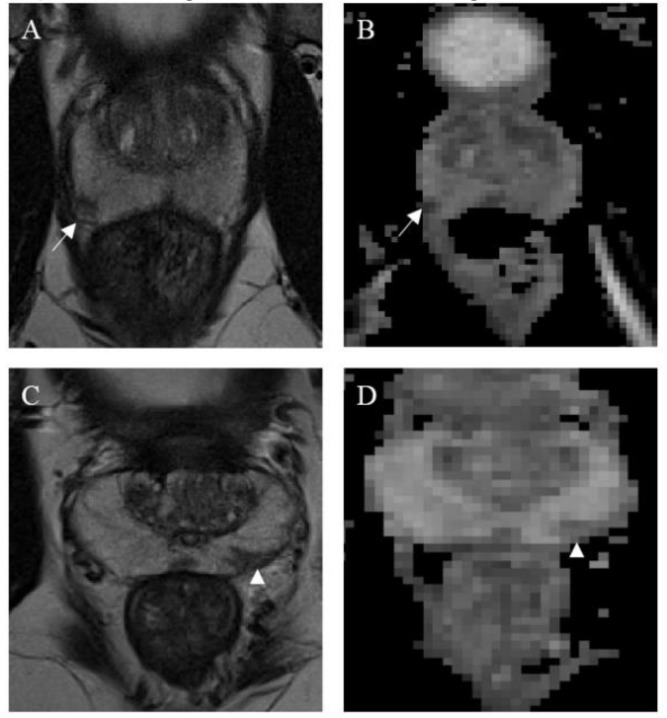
Magnetic resonance images from a clinically significant (A,B) and a non-clinically significant (C,D) prostate cancer lesion. Both are located in the peripheral zone; the clinically significant lesion, found in the right middle-posterior location (white arrows), appears as a nodular and well-defined area of low signal on both T2w (A) and ADC map (B). Conversely, the non-clinically significant lesion, found in the left middle-posterior location (white arrow heads), appears wedge-shaped, with no bulging on T2w (C) and only is mildly hypointense on the ADC map (D). Both lesions were deemed worthy of targeted biopsy.
